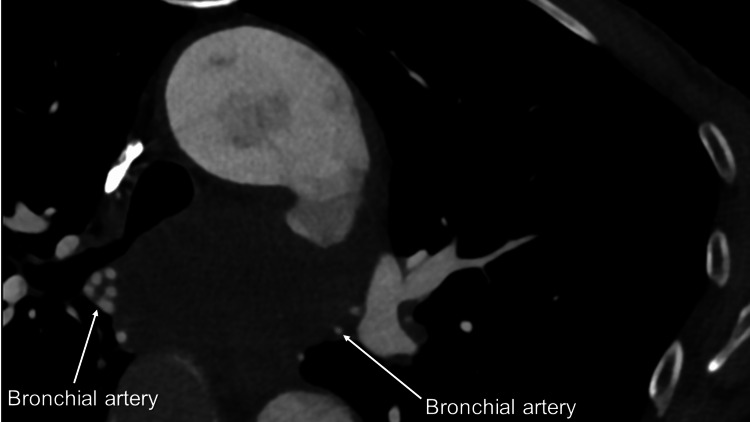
Preoperative contrast-enhanced computed tomography (CT) showing bronchial arteries around a distal arch aneurysm
